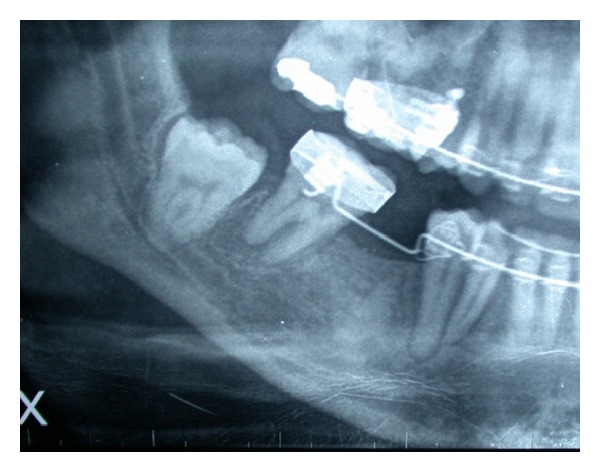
Radiographic view of 46.
